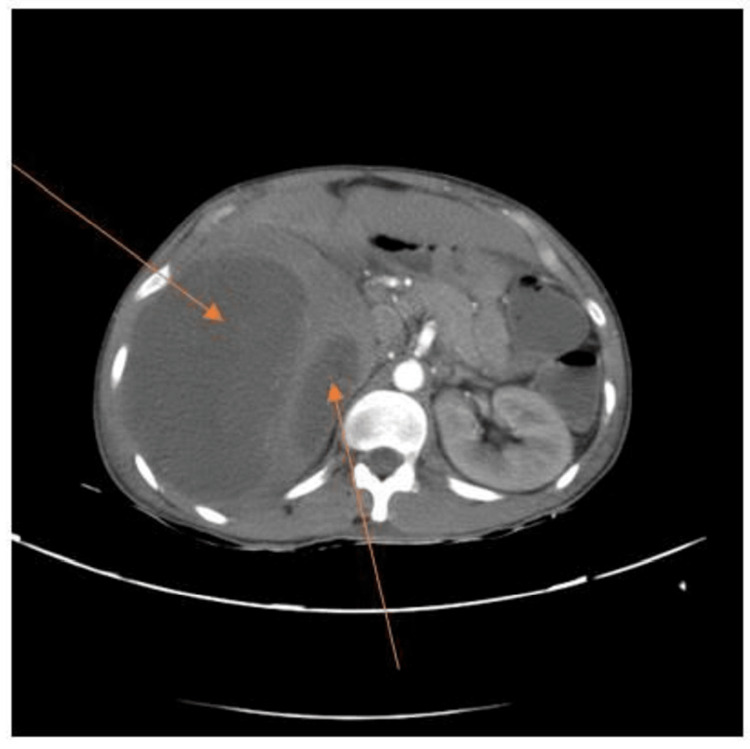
CT scan with IV contrast showing multiple liver abscesses extending from the right to the left lobe of the liver CT: computed tomography
